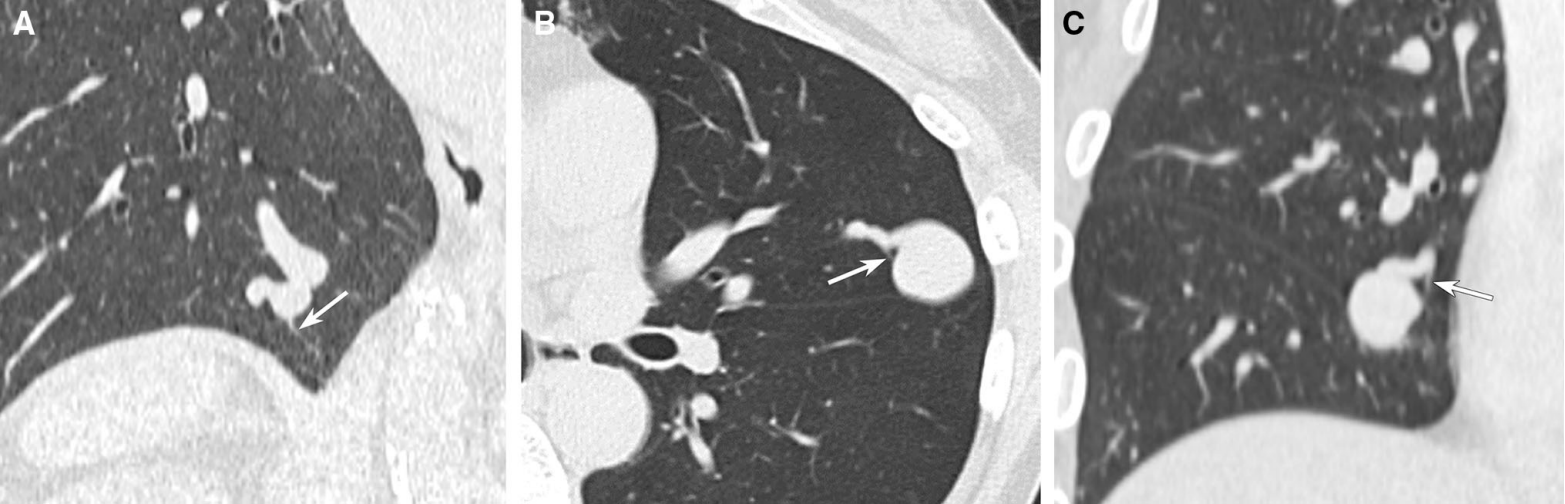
Types of origin of the last normal branch. MDCT images show three portions of the origin of the last normal branches. A The last normal branch originating from “sac”. Oblique coronal MPR CT image shows the last normal branch (arrow) originating from fistulous portion with saccular dilatation. B The last normal branch originating from “junction”. Axial CT image shows the last normal branch (arrow) originating from just proximal portion of saccular dilatation. C The last normal branch originating from “proximal feeder”. Oblique coronal MPR CT image shows the last normal branch (arrow) originating from the proximal feeder, more than 5 mm proximal to the saccular dilatation
